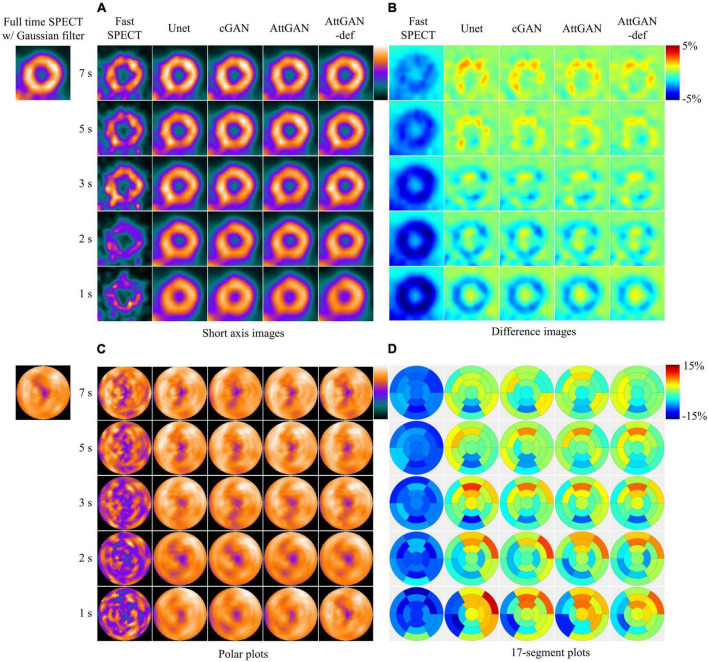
Sample images of a normal male patient (age = 67, BMI = 25.4) before and after DL-based denoising for five shorter acquisition times. The images are shown in (A) short axis images, (B) difference images as compared to FT SPECT, (C) polar plots, and (D) 17-segment plots.
